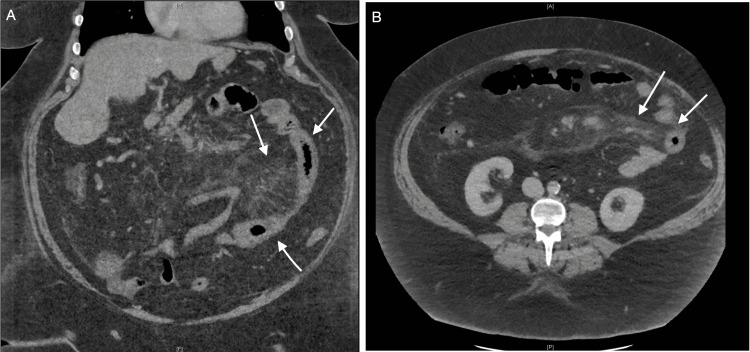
CT showing thickened loops of small bowel suggestive of enteritis and fatty stranding around the mesenteric vessels CT: computed tomography (A) Coronal view. (B) Axial view
